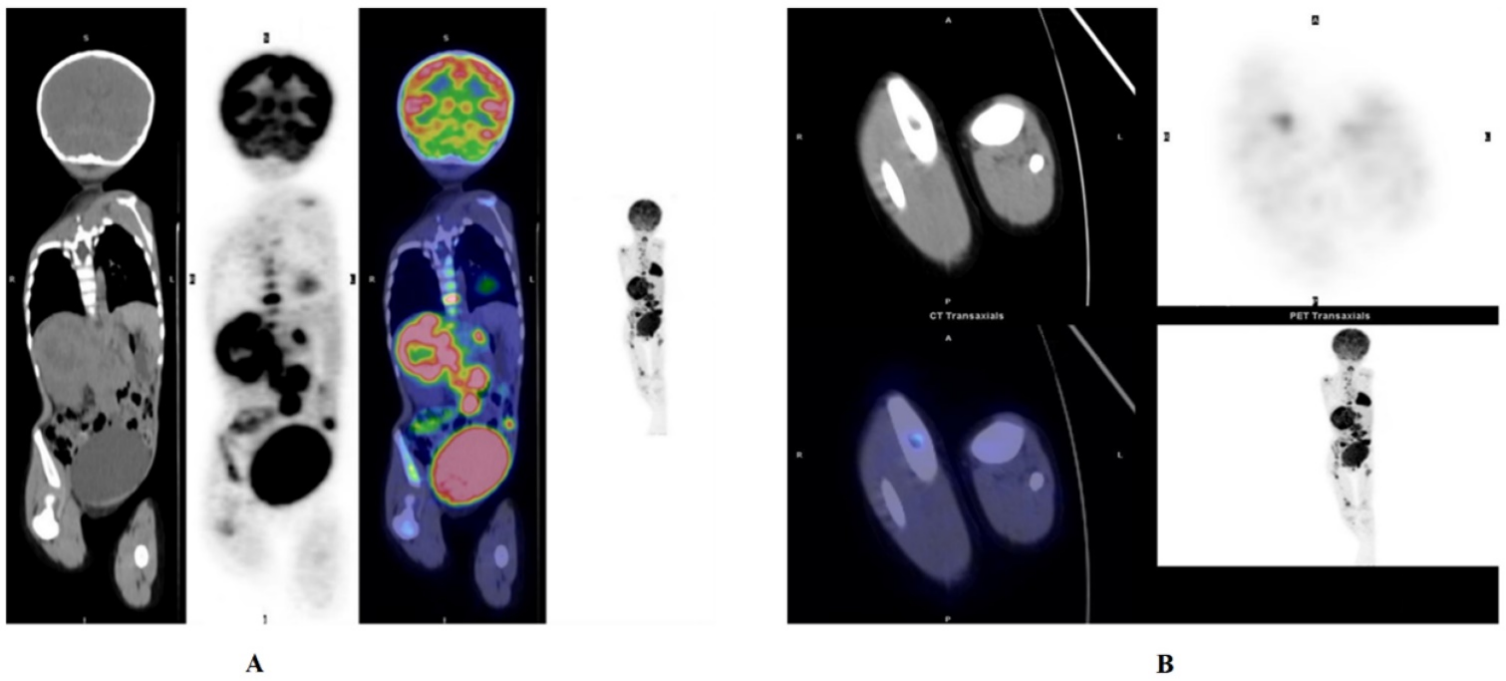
NB. Whole body PET/CT shows NB in the left adrenal gland. Bone marrow infiltration in the left and right femur head as well as the proximal tibia. (A) Activity in the right pelvis was observed. (B)Bone marrow infiltration in the left and right femurs. This patient had a large SUVmax-ratio value, 8.781, and eventually the patient progressed, and eventually died, with very short progression-free survival (PFS) and overall survival (OS).
